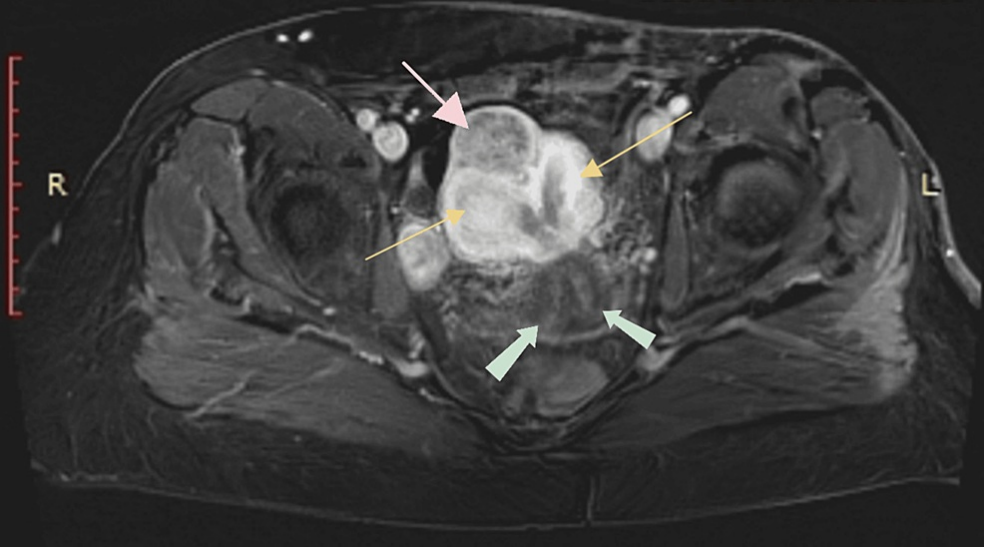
MRI T1-weighted examination showing uterus didelphys (yellow arrows), two cervixes (green arrows), and a uterine leiomyoma (pink arrow - axial plane).
